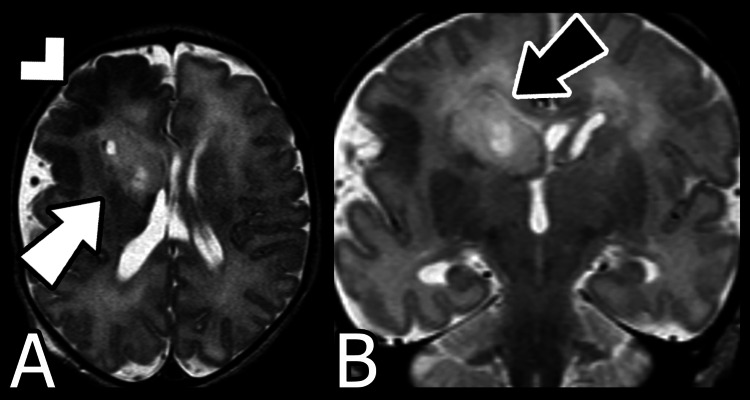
Postnatal MRI on day 3 A) Axial T2-weighted, B) Coronal T2-weighted. The arrowhead emphasizes the severe dysplasia of the right frontal cortex. The white arrow demonstrates dysmorphia of the right basal ganglia and periventricular region with cystic cavities. The black arrow denotes a pointed right frontal horn.
